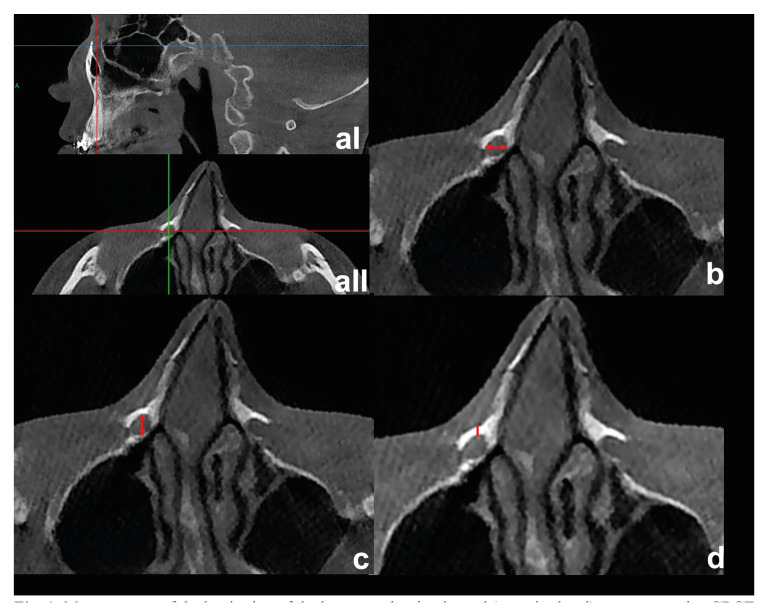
Measurement of the beginning of the bony nasolacrimal canal (superior level) on preoperative CBCT images (aI): CBCT section with standardization before measurements, aII: This section was considered the beginning of the canal, b: The mediolateral diameters of the bony nasolacrimal canal in the section where the canal begins in the axial plane, c: The anteroposterior diameter of the bony nasolacrimal canal, d: The distances between the anterior border of the nasolacrimal canal and the outer cortical surface of the maxilla.
